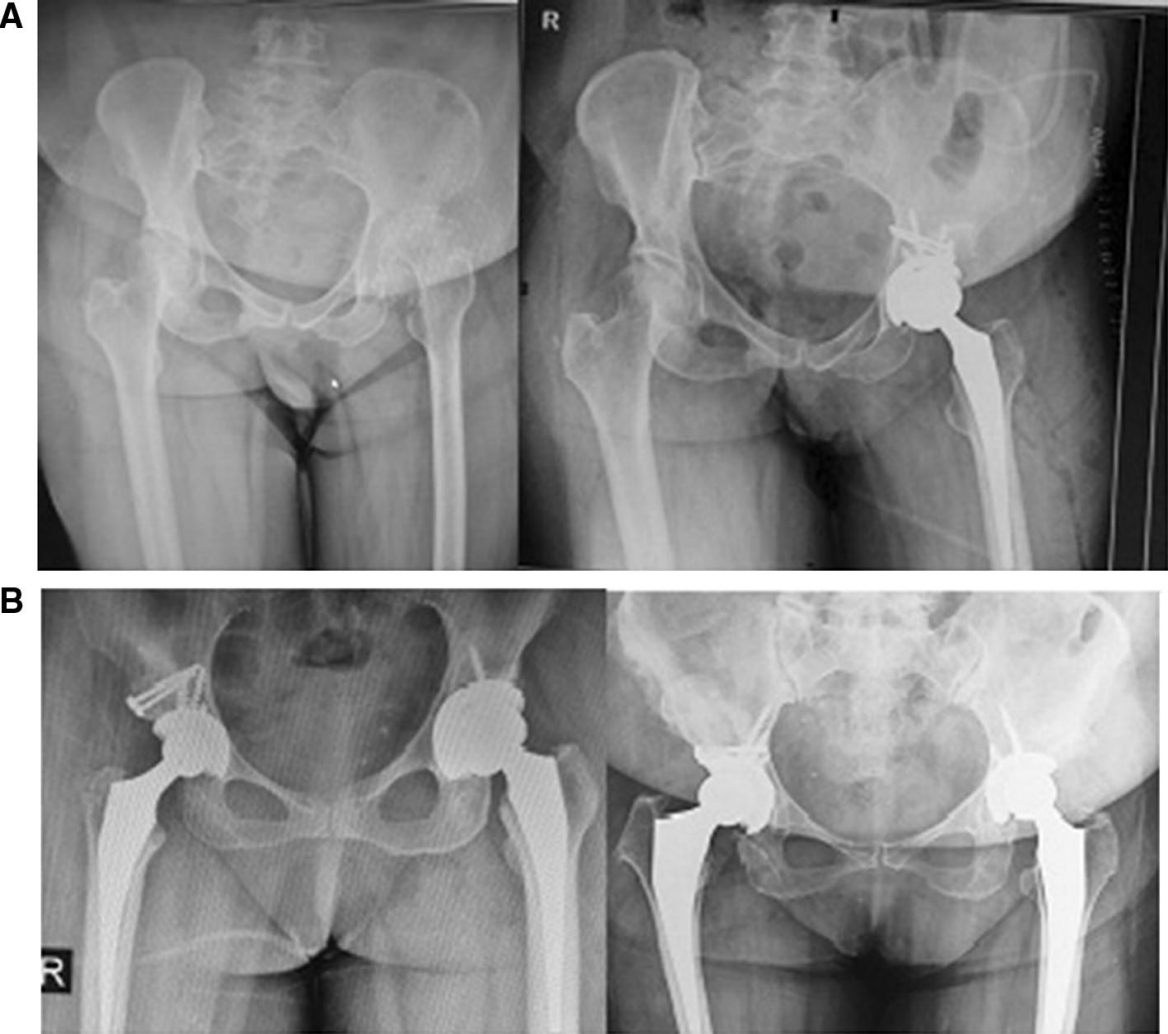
Use of autograft in cases with over than 30% of cup uncovered (a). Postoperative radiographs of two other patients after graft union (b)
